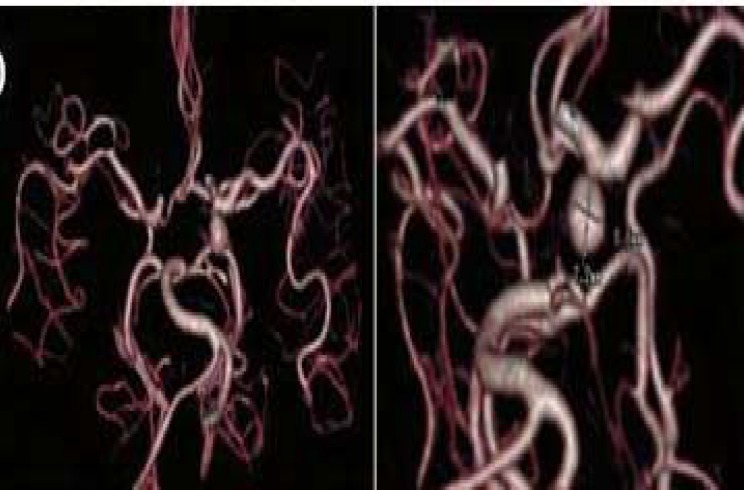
Brain computed tomography angiography revealed an aneurysm in left posterior communicating artery (PCOM)
